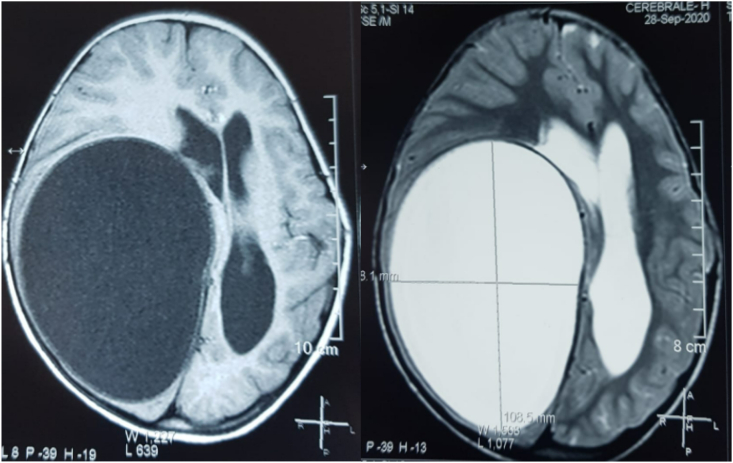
A cerebral MRI was performed showing a rounded hypointense lesion in T1, hyperintense in T2 at the right fronto-temporo-parietal level with an important mass effect and subfalcoral involvement in favor of a cerebral hydatid cyst (Fig. 2).
